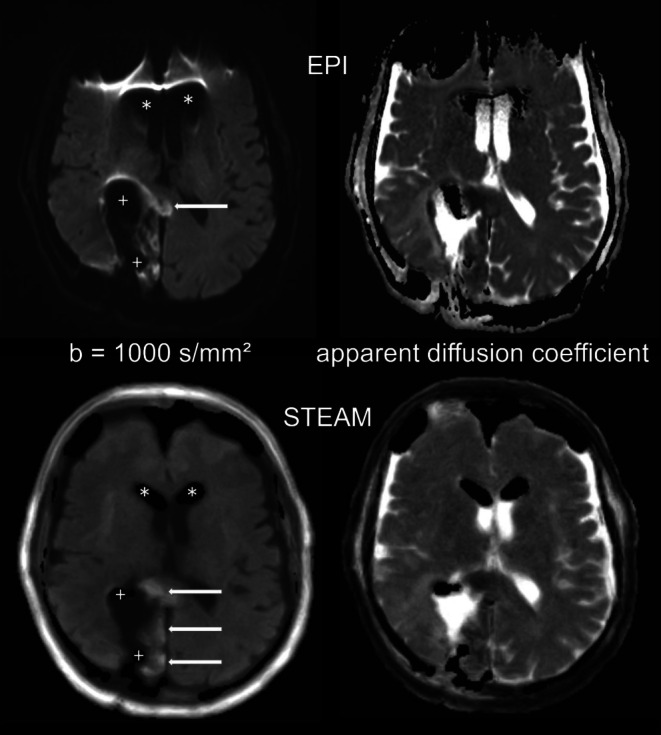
81-year-old patient. Postoperative diffusion weighted images with b = 1000 s/mm^2^ (*left*) and apparent diffusion coefficient (ADC) maps (*right*) obtained by EPI DWI (*top*) and STEAM DWI (*bottom*) 42 h after resection of a glioblastoma of the right parieto-occipital region. The patient presented with trapped air in the ventricular system (*asterisk* ) and the resection cavity (+) and with an infarction of the occipital splenium and the parasagittal occipital lobe (*arrows*)
